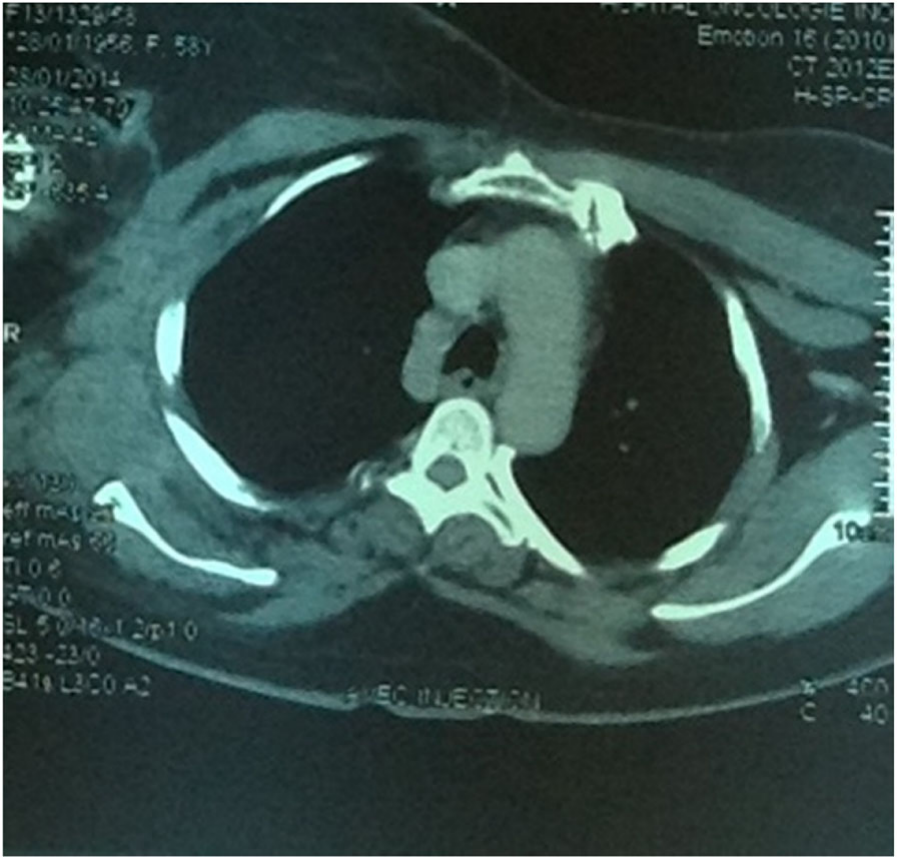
Computed tomography slice showing a mass in the right axillary and subscapularis regions
